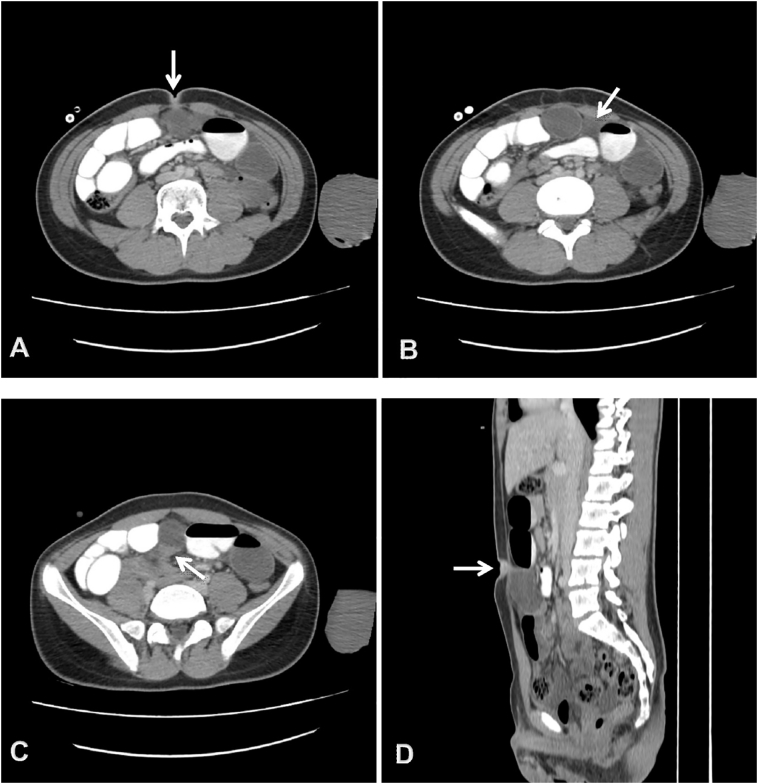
Abdominal CT scan: three sequential axial images from top to bottom (A-C) and sagittal reconstruction (D) demonstrate a midline cyst. The superior aspect seems to be attached to the umbilicus (arrow in A, D) suggestive for omphalomesenteric remnant and the inferior portion is in contact with small bowel loops (arrow in C) depicting mechanical obstruction. Dilated proximal small bowel loops are opacified. Small volume of interloop fluid is present (arrow in B).
